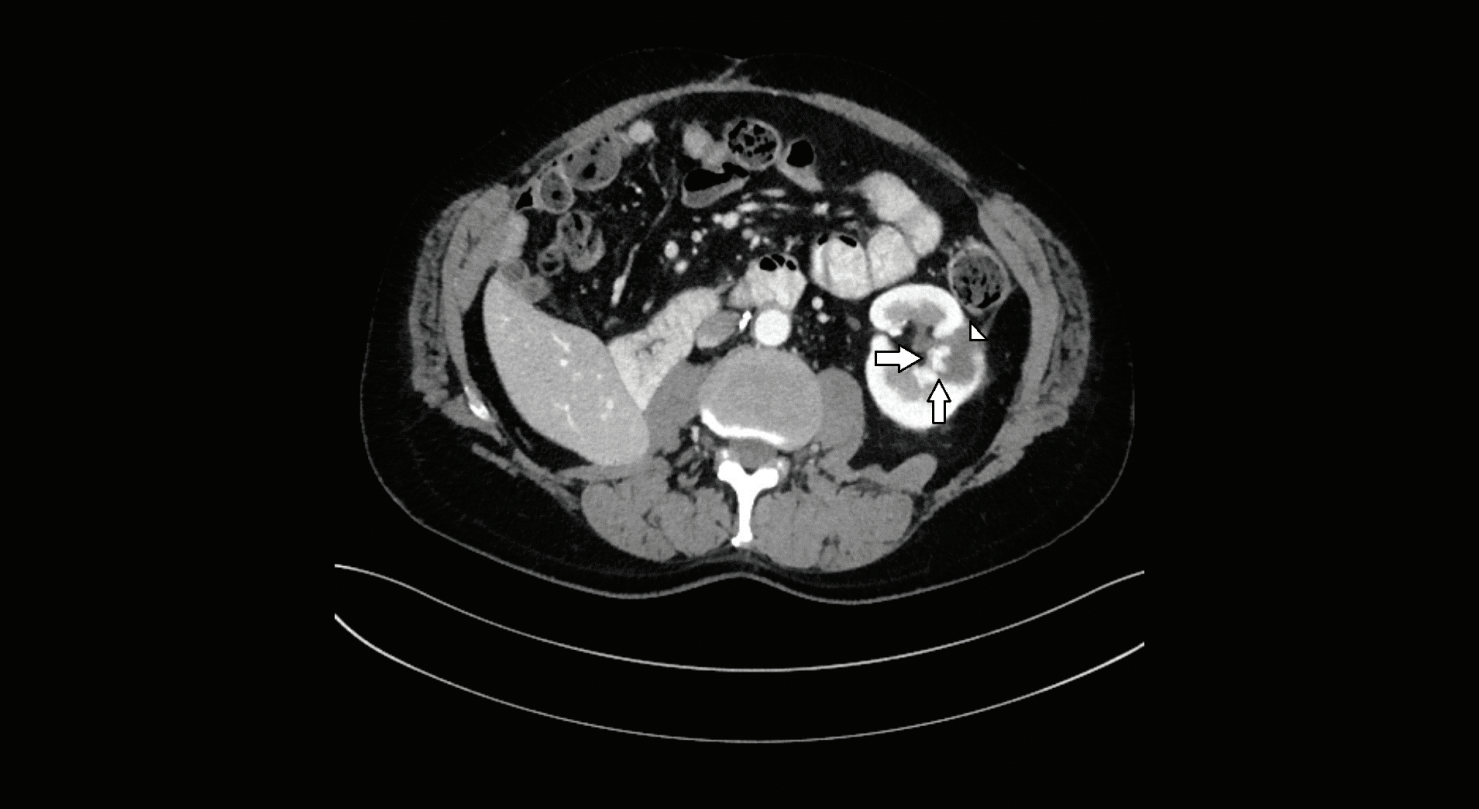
Axial CT image shows the hypervascular nodule (arrows), centrally located, on the inner margin of the previous ablation area (head of arrow).
